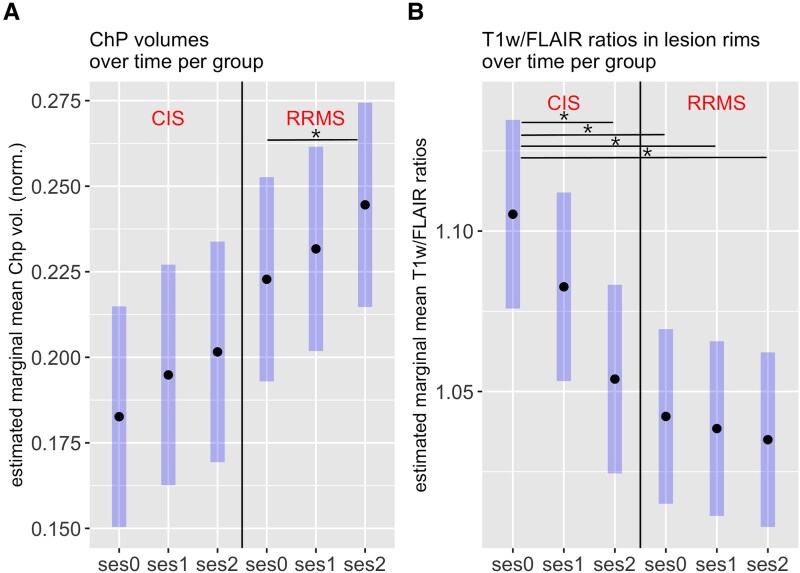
Longitudinal biomarker patterns for CIS and RRMS patients. Time courses of ChP volume (normalized to intracranial volume) (A) and T1w/FLAIR ratios in lesion rims (B) for CIS (*N* = 18, left panel) and RRMS (*N* = 22, right panel) patients. Values were derived from linear mixed model analysis and represent estimated marginal means for baseline (ses0), follow-up after 2 years (ses1) and follow-up after 6–8 years (ses2) as bold dots and 95% confidence intervals as highlighted bars. The analysis included age (at baseline) and sex as fixed effects and subject as random effect. Significant differences (*P* < 0.05) are marked with asterisks.
